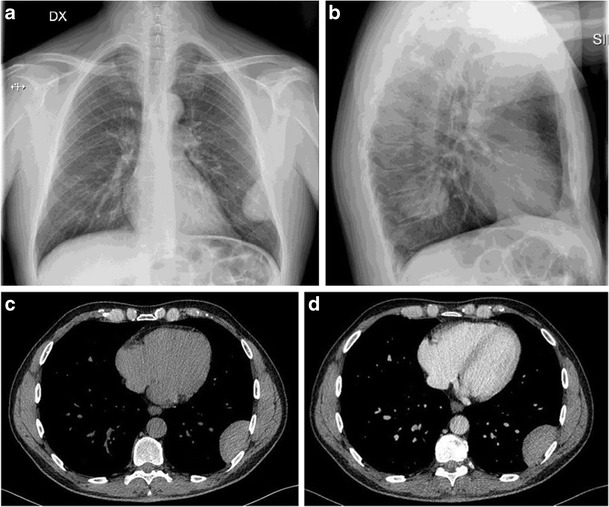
An accidental case of solitary fibrous tumour in a 56-year-old man: standard chest radiography (a, b) demonstrates a well-defined, ovalar shaped chest wall mass. Contrast-enhanced CT, axial plane before (c) and after (d) contrast media administration showing a bulky, homogeneus and non-enhancing mass of the left posterior chest wall
